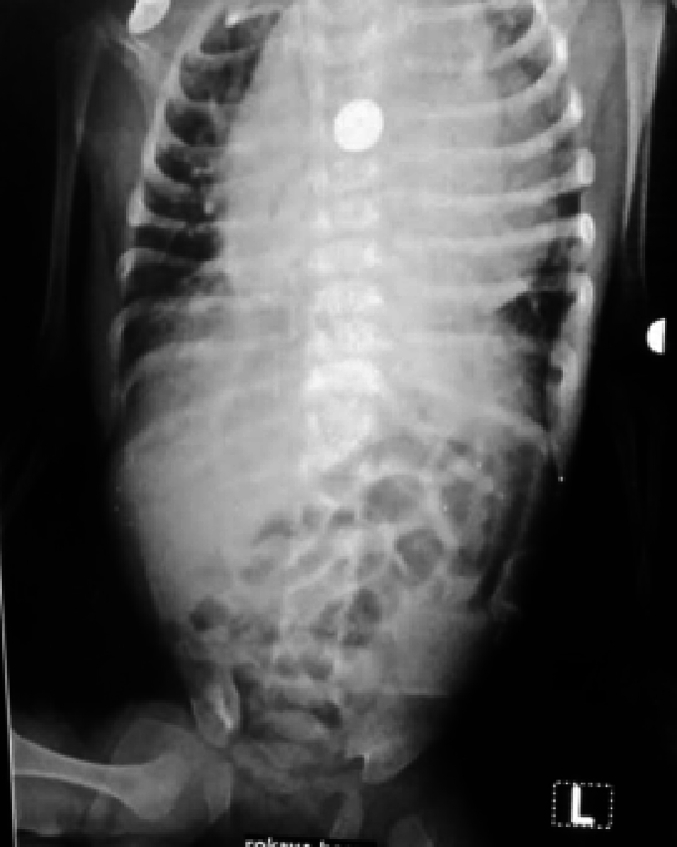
Preoperative echocardiography showed cardiomegaly.
